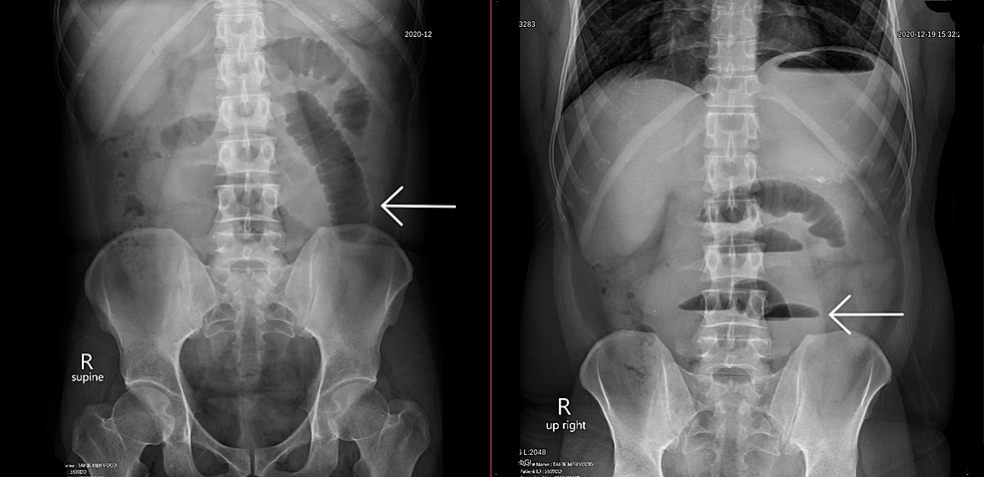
Abdominal X-ray. X-ray demonstrates gas-filled small bowel loops (Left) with numerous air-fluid levels (Right), suggesting small bowel obstruction.
